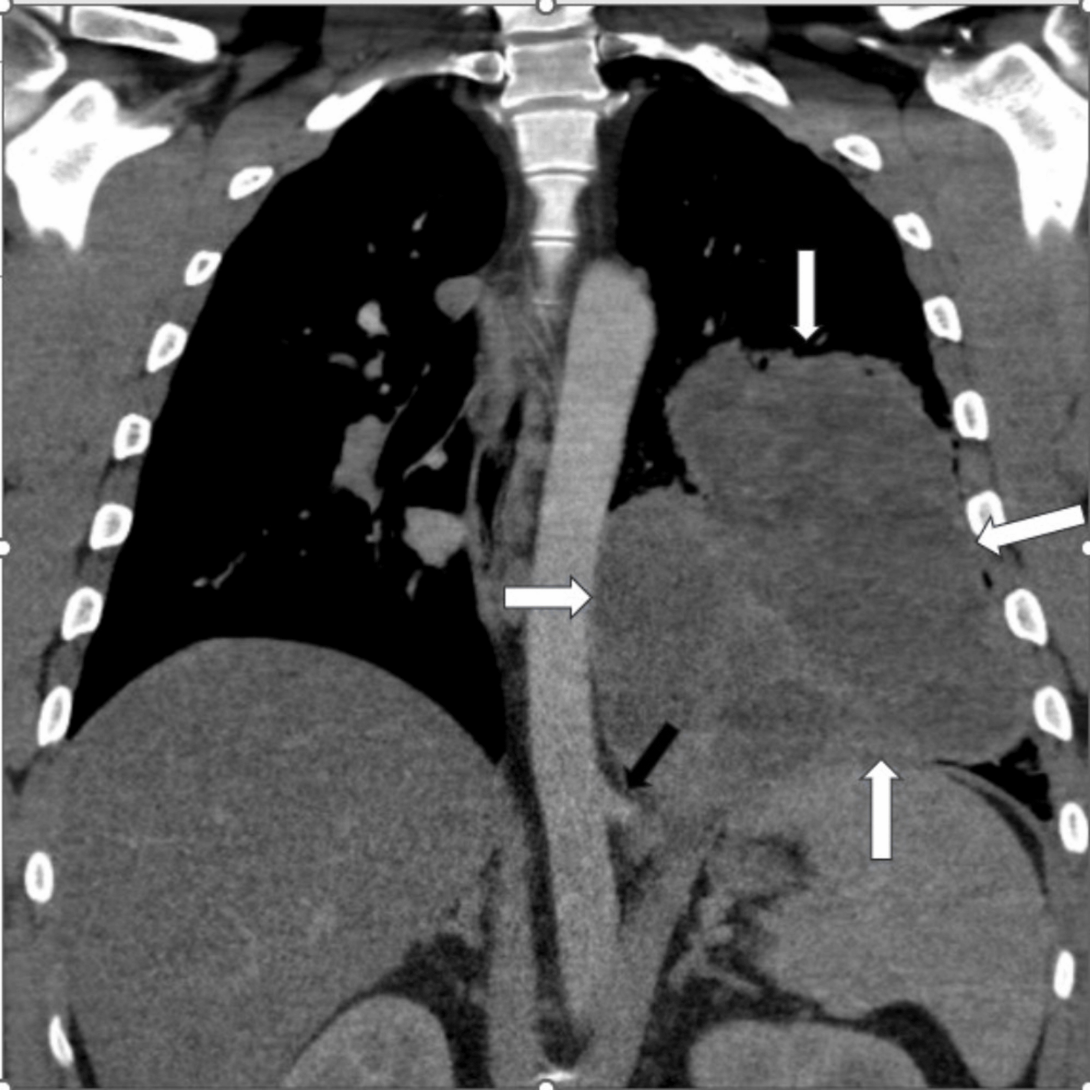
Admission chest CT scan Coronal contrast-enhanced pulmonary CT angiogram shows a large low attenuation lesion measuring fluid density with enhancing septations (long white arrows). There is partial visualization of an aberrant vessel arising from the thoracic aorta (long black arrow).
